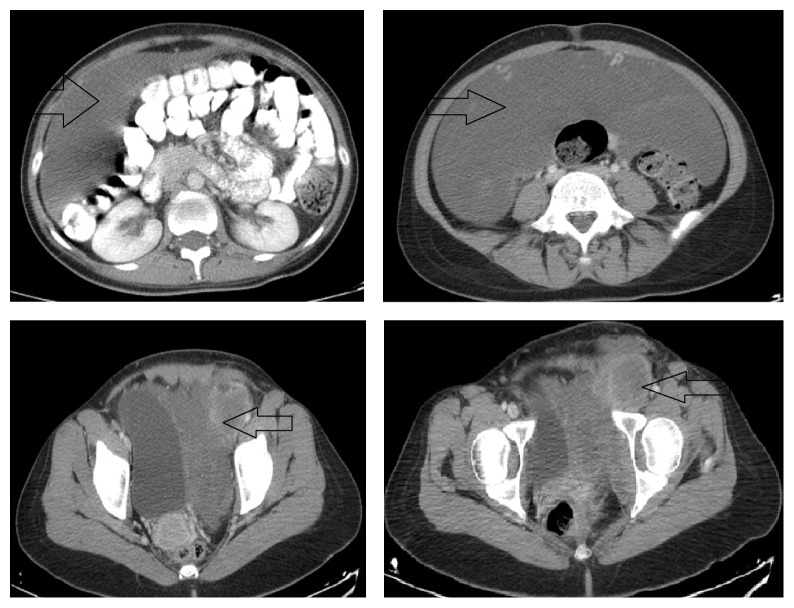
Computed tomography findings of the tumor.
